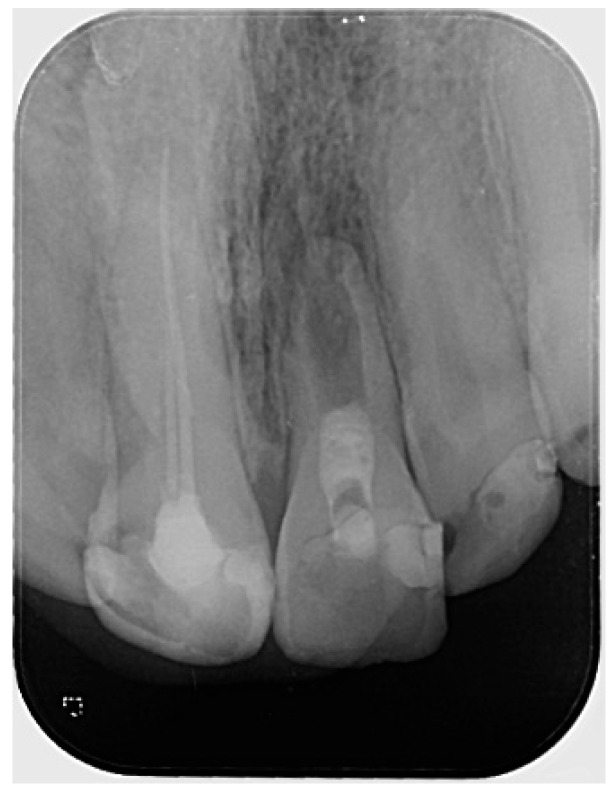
Preoperative radiograph of tooth #21. The radiograph shows previous endodontic treatment, with the root canal filling material not extending to the full length of the canal. A diffuse radiolucency is visible around the open apex. (The mark in the lower left corner indicates the left side).
